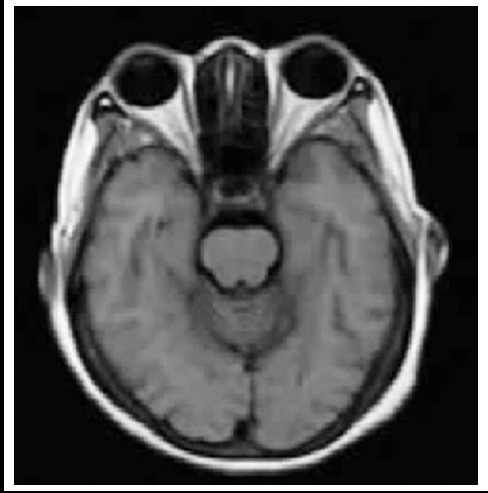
MRI plain scan images of viral meningitis (mainly menifested as multiple and irregular lesions with abnormal long-T1 and T2 signals)
